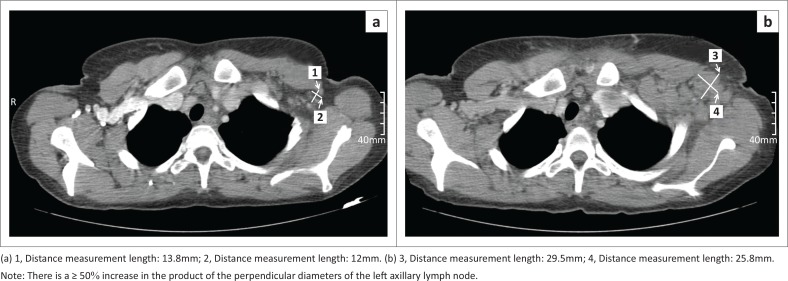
Progressive disease in Hodgkin’s lymphoma, initial computed tomography (CT) (a) and restaging CT (b).
